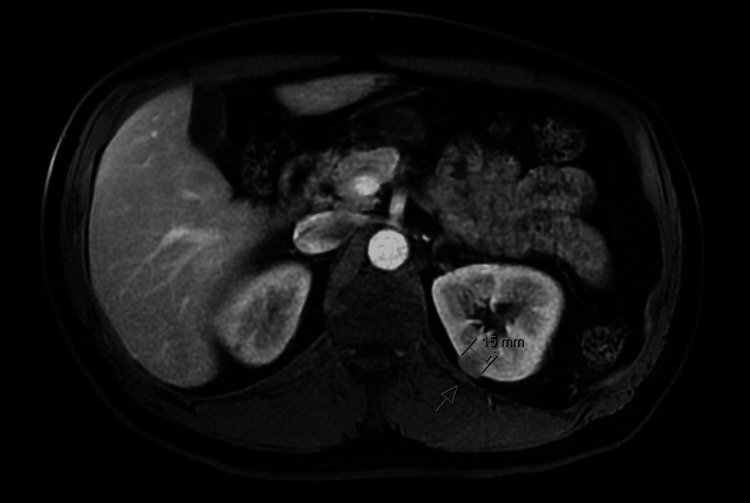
MRI of the abdomen showing renal mass (arrow)
